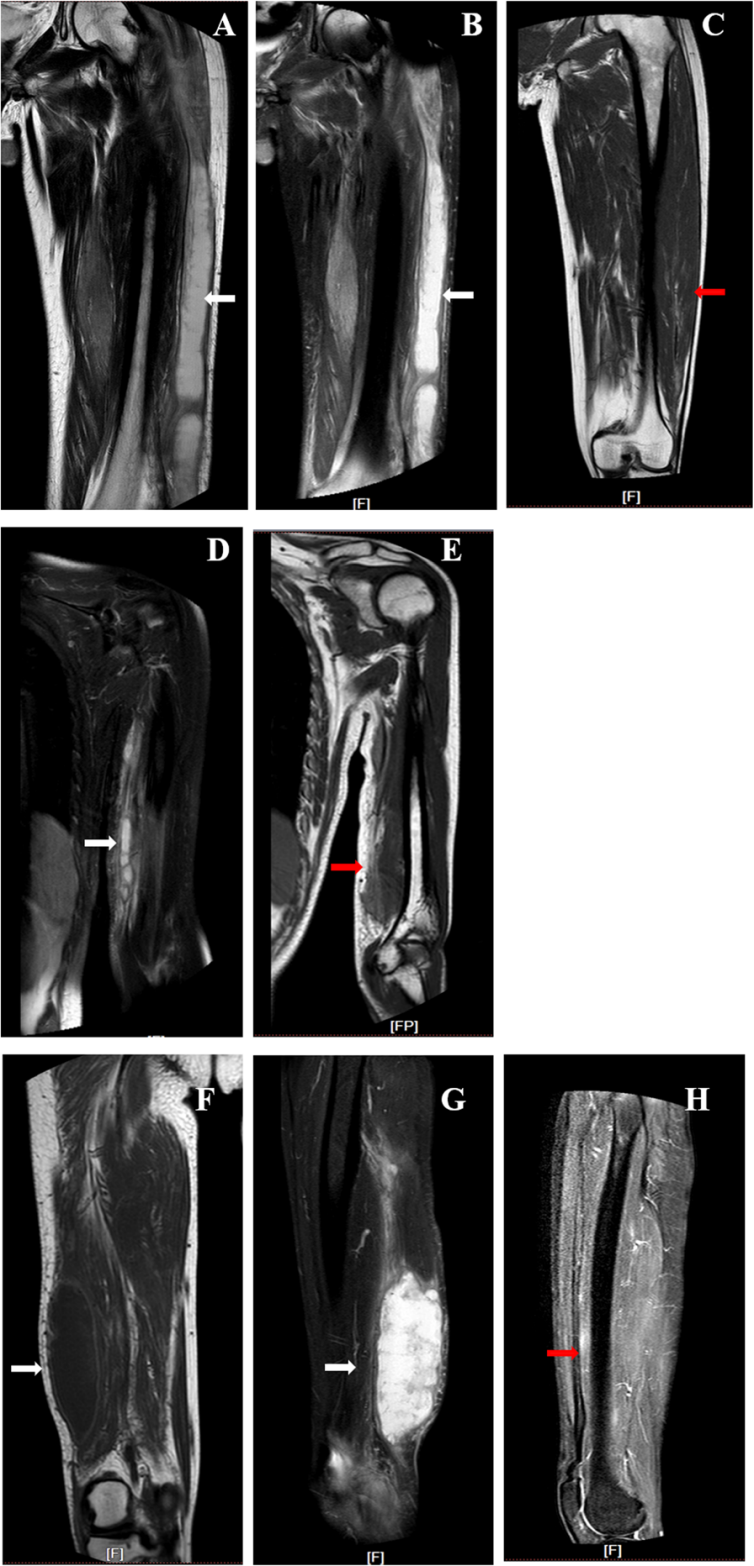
MRI of subcutaneous abscesses in the limbs pre and post treatment. MRI of the left femoral showed two different fluid collections extended along the path of subcutaneous connective tissue (upper panel, A and B). There was a spot with slightly high signal at the lower end of the left humerus (middle panel, D). After the comprehensive treatment, the left femur and the left humerus abscesses faded away obviously (C and E). Another mass was on the right femur above the right popliteal fossa, without fluid fluctuation (lower panel, F and G). After surgical removal of the abscesses, the abscess lesion on the right humerus was restored (H)
